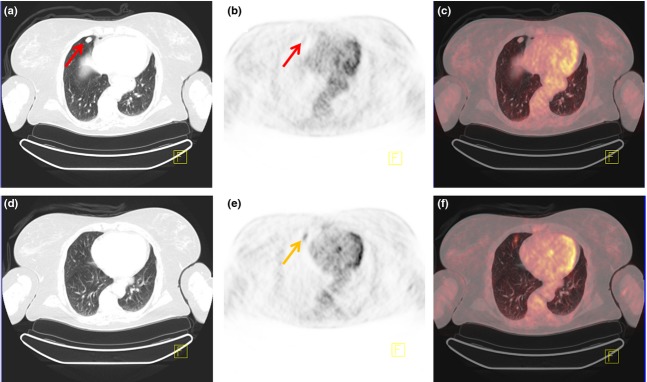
PET/CT scan of a 60-year-old female with a tumour anterior in the right lung, easily visible on CT (a). However, on PET/CT, FDG uptake was hardly visible in the area of the tumour (b,c). Taking a closer look at the slices above the tumour (d–f), moderately focal increased FDG uptake was visible here (SUV_max_ = 4). This uptake corresponds to the tumour, but as a result of respiratory movements during PET acquisition, there is a misalignment between CT and FDG-PET. The patient was diagnosed with a carcinoid tumour, which explains the relatively low FDG uptake.
